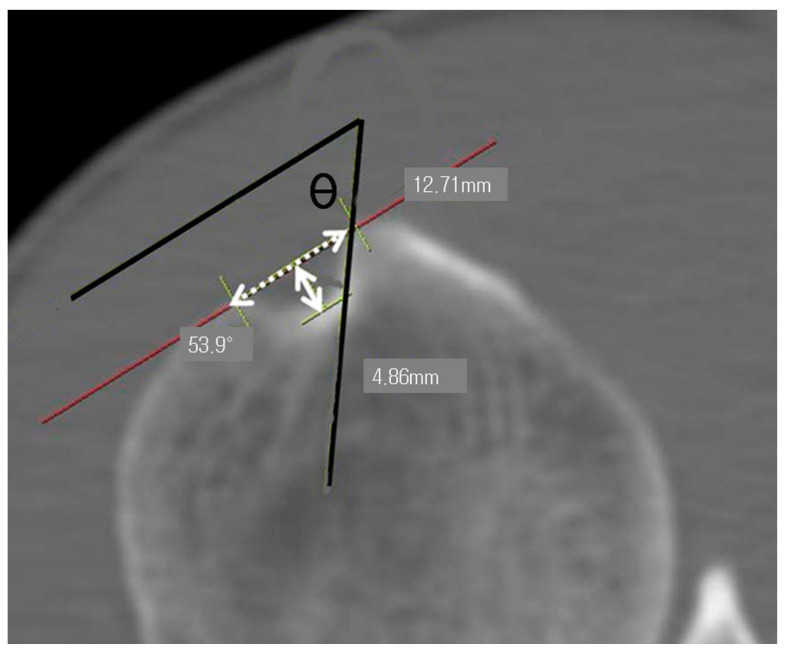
The axial CT image shows the parameters of the bicipital groove. The solid arrow indicates the depth, while the dashed arrow indicates the width of the groove. The angle (θ) represents the medial wall angle, formed by two solid lines: one along the medial wall and the other parallel to the line connecting the highest points of the medial and lateral walls.
